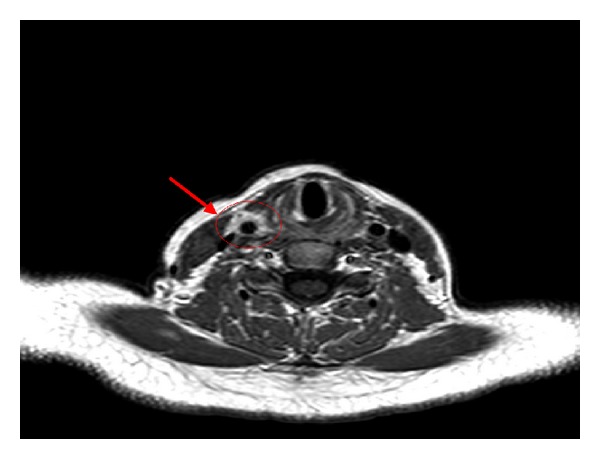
Magnetic resonance (MR) imaging of the neck. MR shows localized enhancement in T1-weighted images involving right medial-distal common carotid artery (arrow); the thickening of perivascular soft tissue might be the result of localized vascular inflammation.
